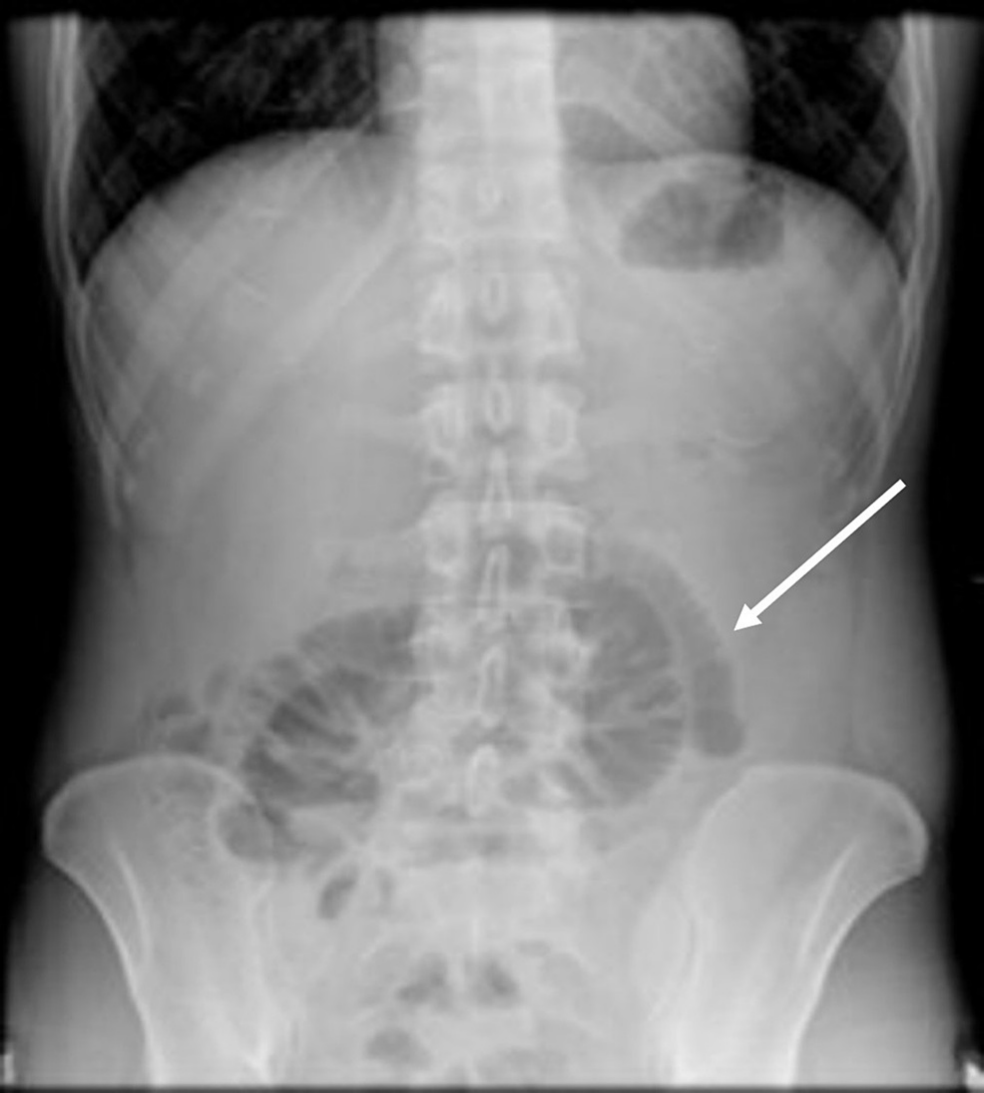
Air-fluid levels on X-ray showed a small intestinal obstruction.
